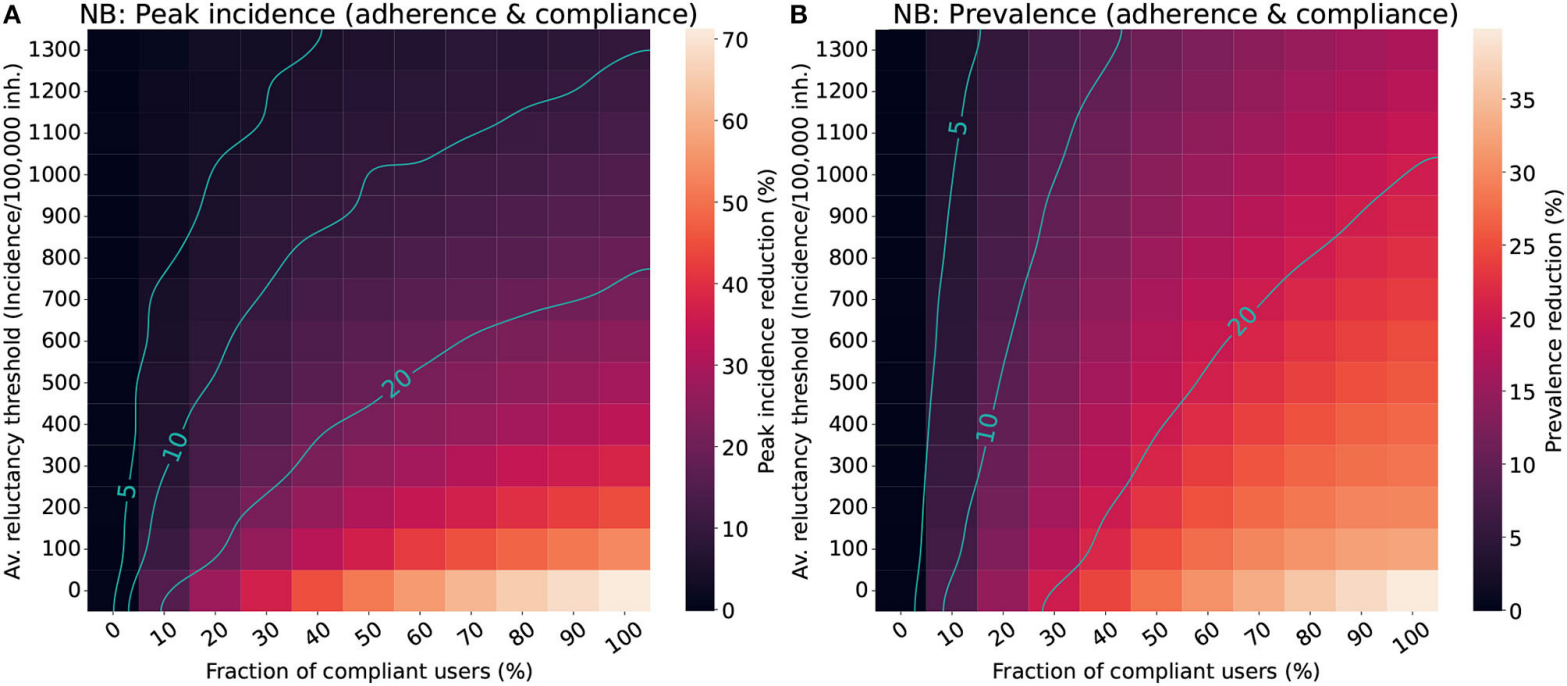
Impact of the population's average reluctance threshold and the level of compliance in the effectiveness of CT apps for a population with a Negative binomial distribution (NB). We assumed a maximal fraction of adopters of 70% for the “adherence & compliance” scenario. The color scale reflects the average reduction produced by the CT app (Δ) in the peak incidence (A) or maximal prevalence (B). The isoclines indicate the combinations of parameters resulting in Δ = 5%, Δ = 10%, and Δ = 20%.
